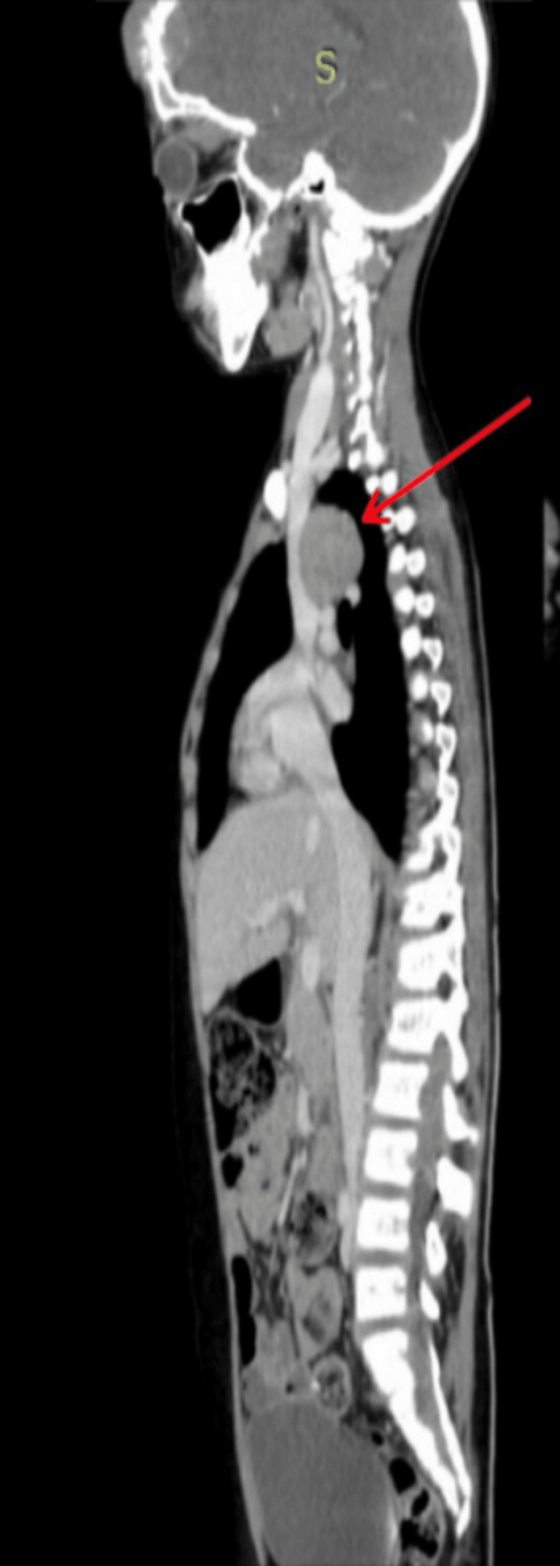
Contrast-enhanced CT showing lesion compressing the esophagus posteriorly.
